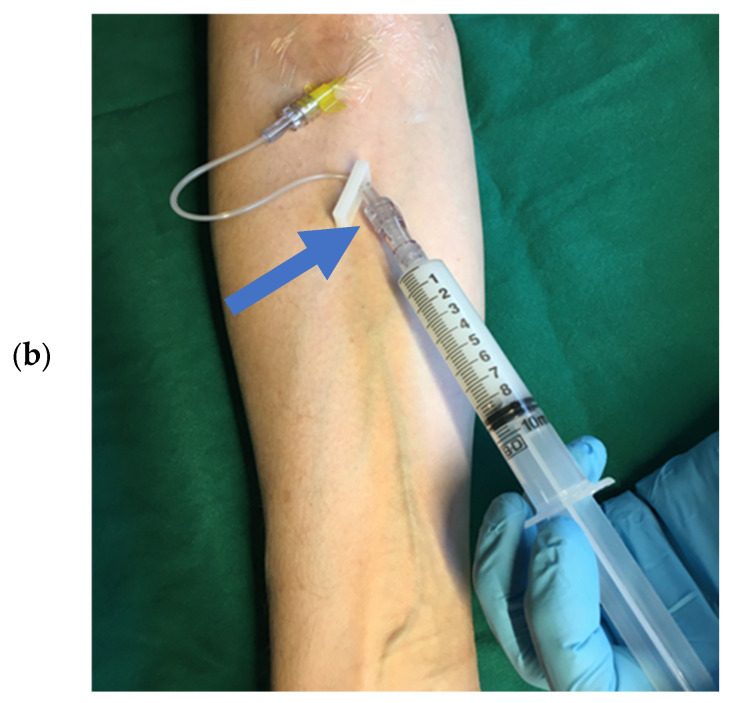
(a) Injection of UEAs with a flush via a three-way stop cock. For re-agitation, the syringe with the diluted UEA must be removed from the stop cock and tilted several times. (b) Injection of UEAs without a flush via a short extension line that connects the IV line with the 10 mL syringe, as well as the microclave, clamp, and rotating luer. For re-agitation of the diluted UEA, the echocardiographer picks the syringe at the luer connector (arrow) and tilts the syringe forward and backward. This way, there is less risk of pulling the IV, and the system remains closed.
